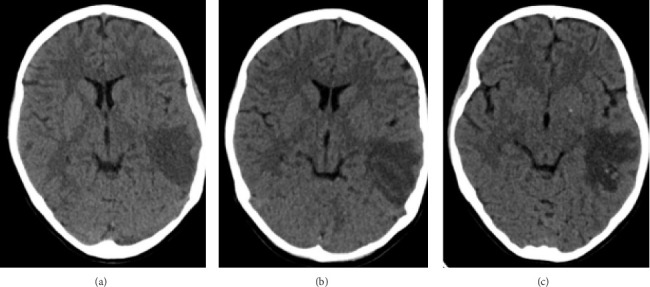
CT brain without contrast enhancement taken on Day 1 (a), Day 4 (b), and Day 10 (c) of admission, respectively. There was an extensive white matter hypodensity in the left temporal lobe on plain CT brain on admission (a) with mild enlargement on Day 4 of admission (b). There was no intracranial hemorrhage on initial presentation. The interval scan on Day 10 of admission (c) showed new hyperdensities with the hypodensity in left temporal lobe, which may be compatible with hemorrhagic component.
